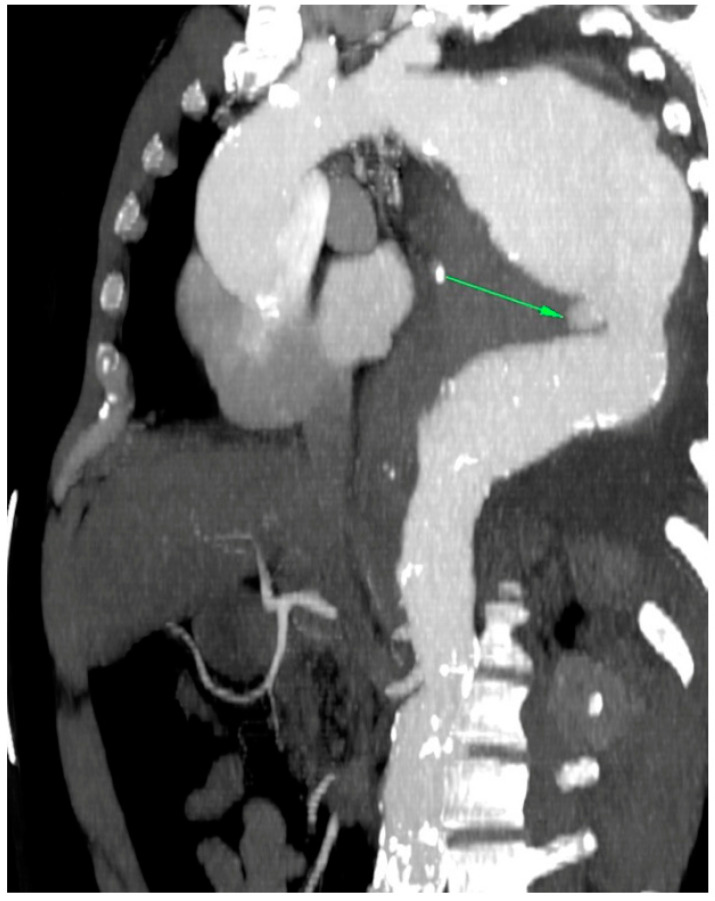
CT-scan of a thoracic aortic aneurysm rupture with an aortoesophageal fistula (green arrow).
